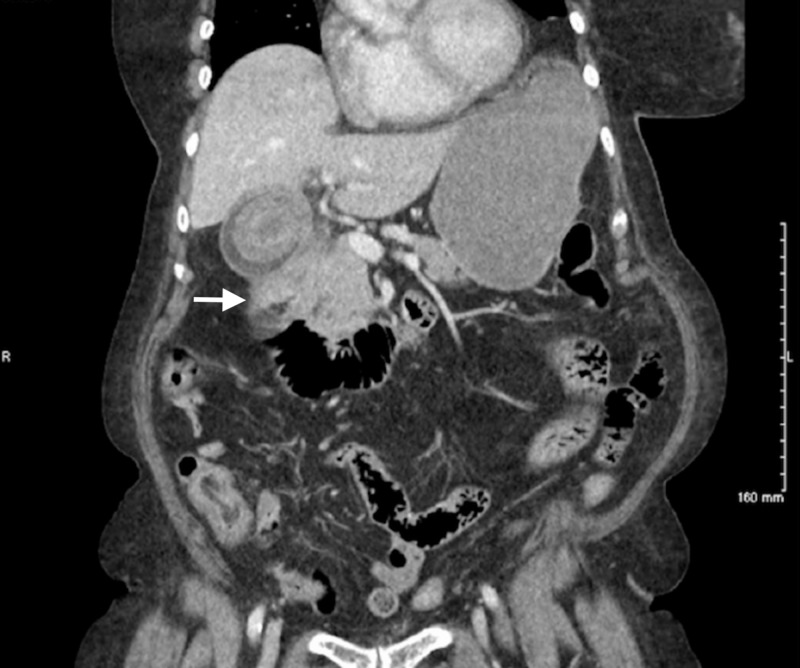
Computed tomography scan obtained one year after initial presentation showing extensive duodenal inflammation (white arrow).
